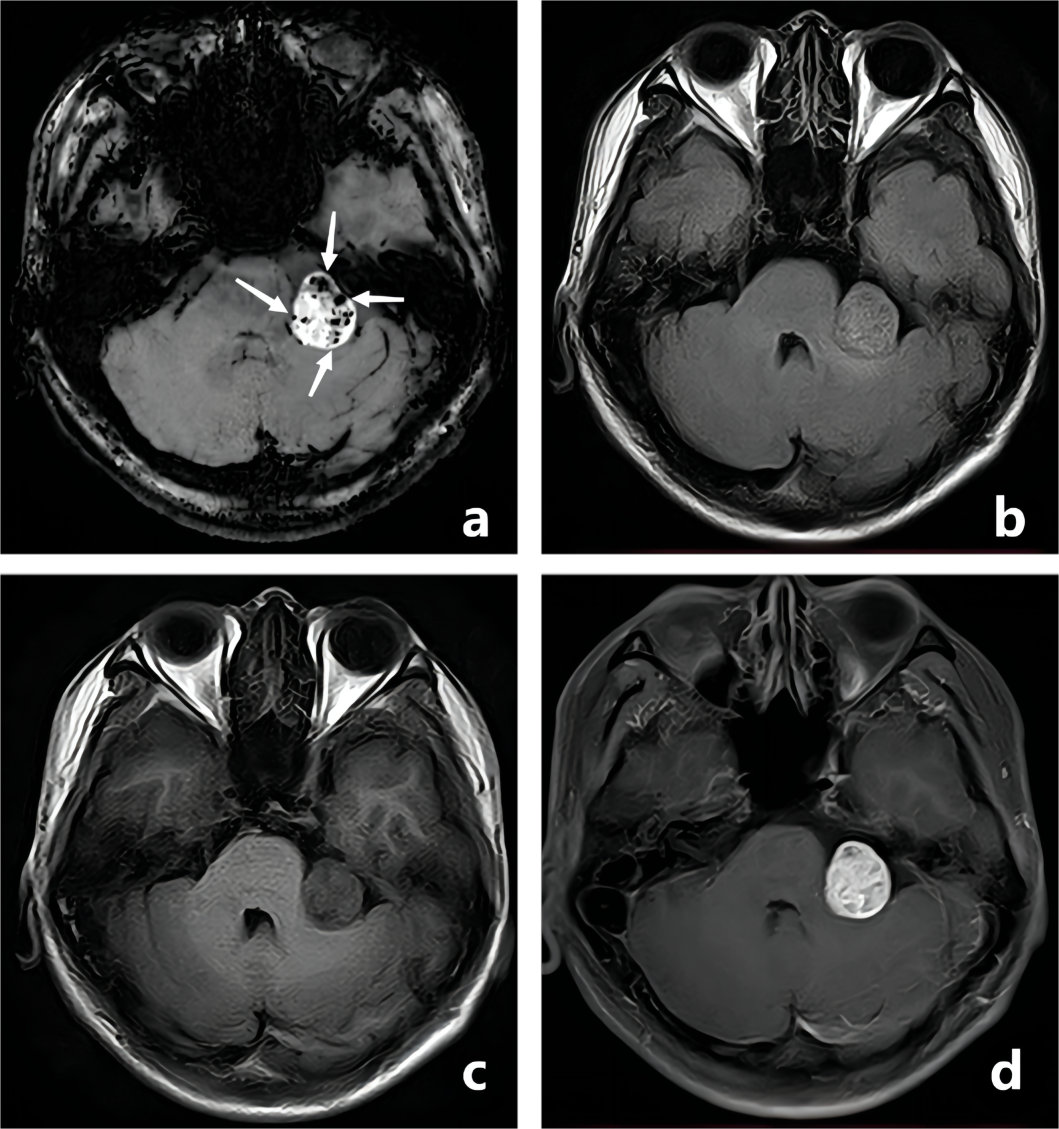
A-28-year-old man with a pathologically confirmed schwannoma in his left cerebellopontine angle area after surgical resection. The schwannoma showed obvious inhomogeneous enhancement, and the intratumoral microbleeds showed speckled low signals on CE-T_1_WI FSBB (A, white arrows). Microbleeds were not clearly visible on FLAIR (B), T_1_WI (C), and CE-T_1_WI (D).
